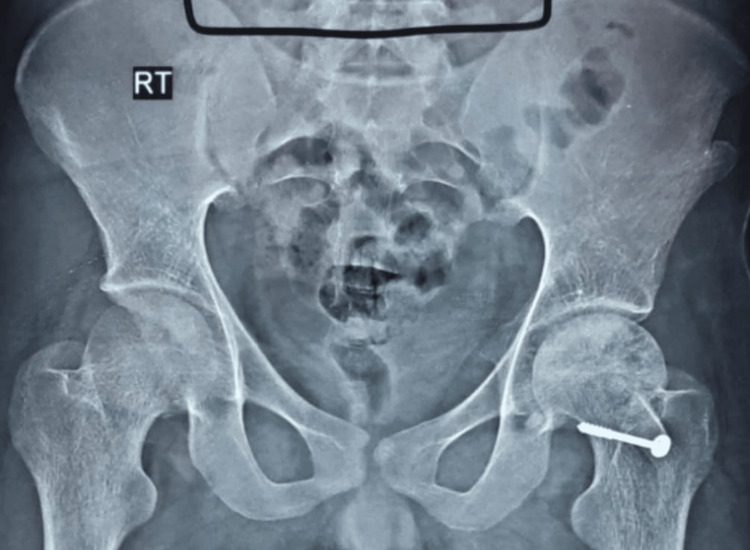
Postoperative radiograph at six months of follow-up
